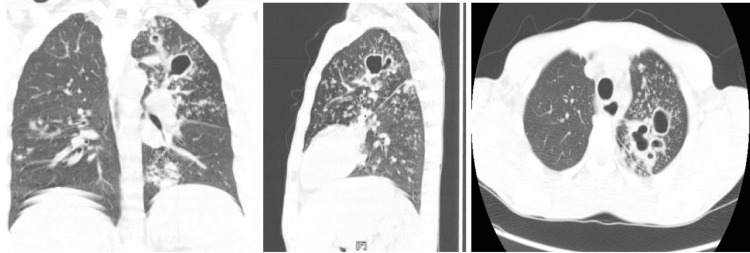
CT of the chest showing left upper lobe cavitations CT = computed tomography
